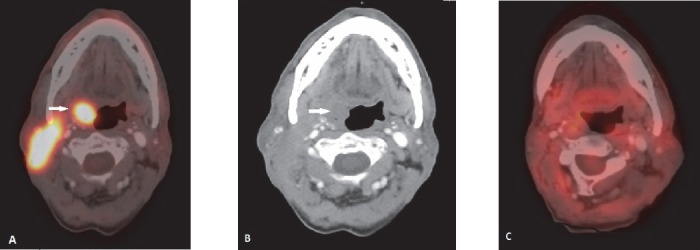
NHL involving tonsil: Asymmetric radio tracer uptake in right tonsil (arrow in A). CT scan shows fullness in right tonsilar fossa (arrow in B). Metabolically active enlarged right cervical lymph node is also seen. Post 3 cycle chemotherapy shows complete metabolic response (C)
